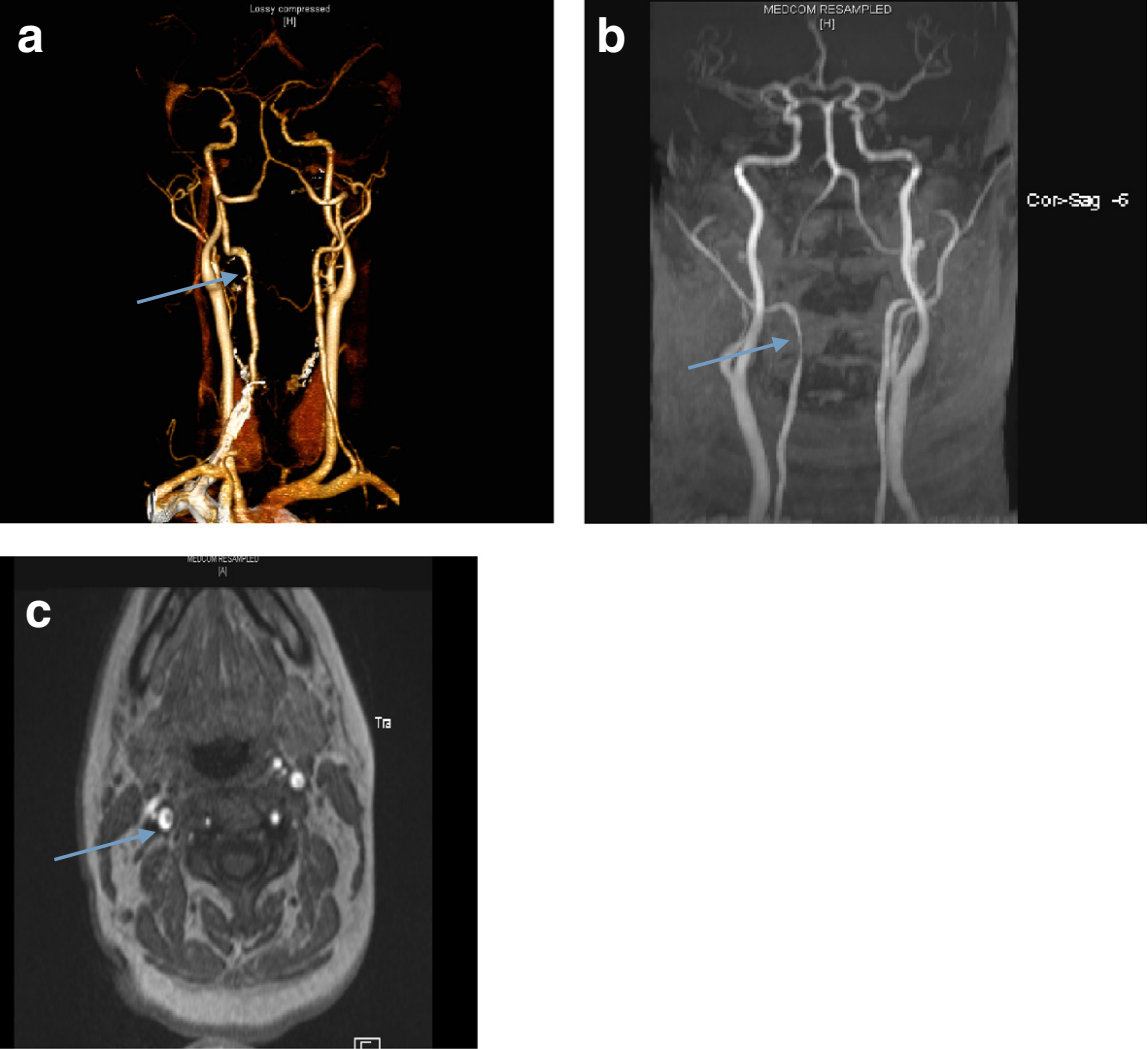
a CTA Neck : Focal stenosis with thickened wall and an intramural thrombus of left vertebral artery at the level of C3 (arrow). b and c A MRI coronal (b) and horizontal (c) view of the vertebral arteries confirms a 1.1 cm C2-C3 right vertebral artery dissection with no evidence of posterior circulation cerebral infarct (arrow)
